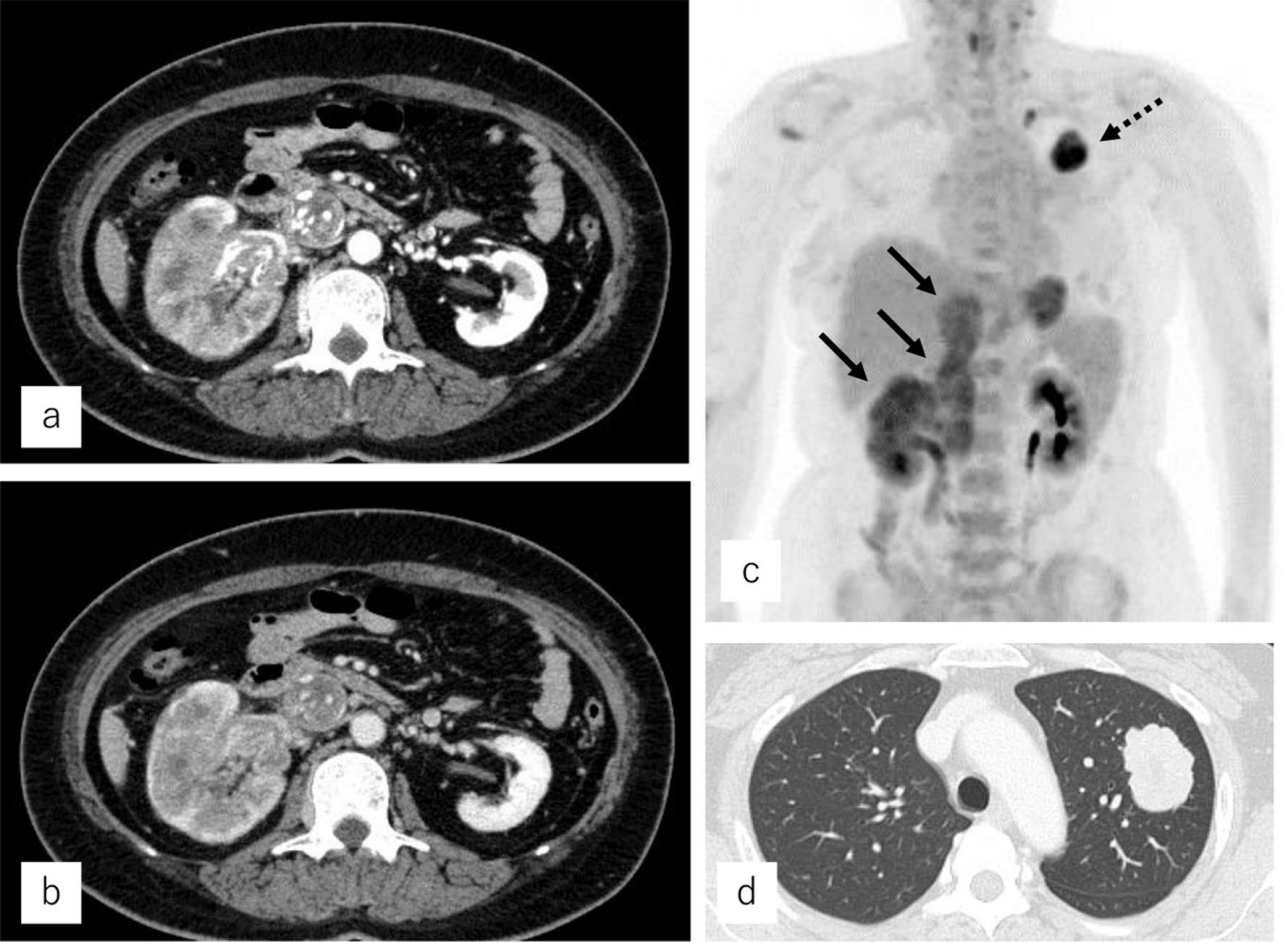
Contrast-enhanced CT. A right renal tumor is occupying the entire right kidney and protruding outside the cortex. There is a tumor thrombus visible in the inferior vena cava on early phase (a) and late-phase (b) imaging. Positron emission tomography–CT shows kidney metastasis with a tumor thrombus in the inferior vena cava (arrows) and lung metastases (dashed arrow) (c). Plain CT shows a tumor in the left lung (d)
